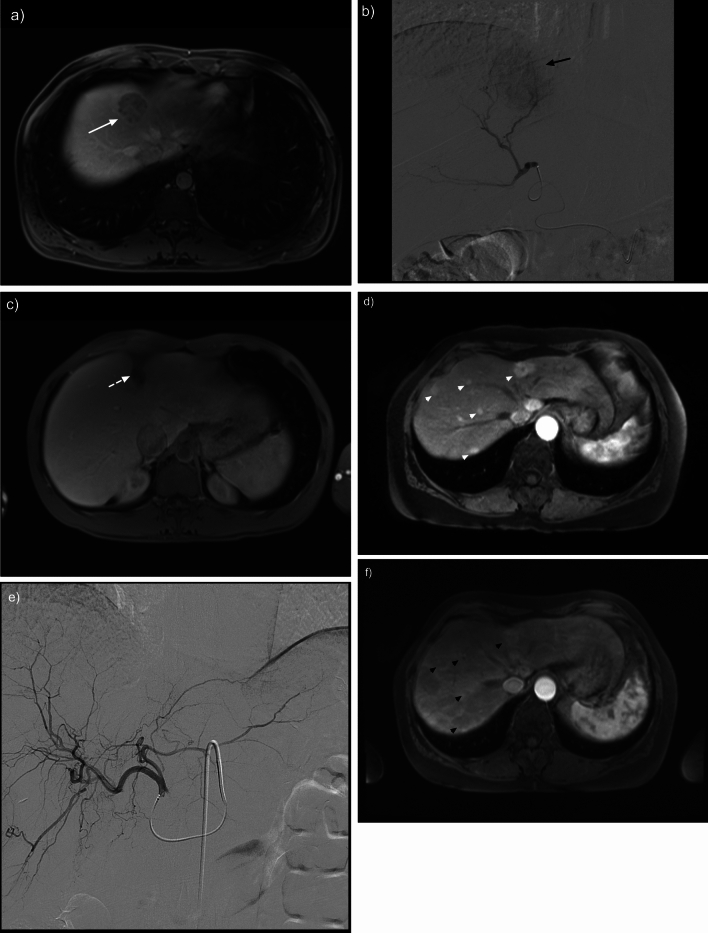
Representative images of index tumors status post ^90^Y radioembolization. Index tumor segmentectomy: a T1 weighted contrast enhanced MRI demonstrating an enhancing lesion in segment IV (white arrow). b Representative angiogram demonstrating ^90^Y segment radioembolization (black arrow). c T1 weighted contrast enhanced MRI demonstrating complete radiographic response of the treated lesion 5 years later (white dotted arrow). Index tumor lobar treatment: d T1 weighted contrast enhanced MRI demonstrating multiple hypervascular tumors in the right hepatic lobe (white arrowheads). e Representative angiogram image demonstrating right sided ^90^Y lobar radioembolization. Note, there is a replaced right hepatic artery arising from the superior mesenteric artery. f T1 weighted contrast enhanced MRI demonstrating partial radiographic response 3 months later (black arrowheads). This patient later underwent additional ^90^Y lobar radioembolization treatments of the left hepatic lobe
